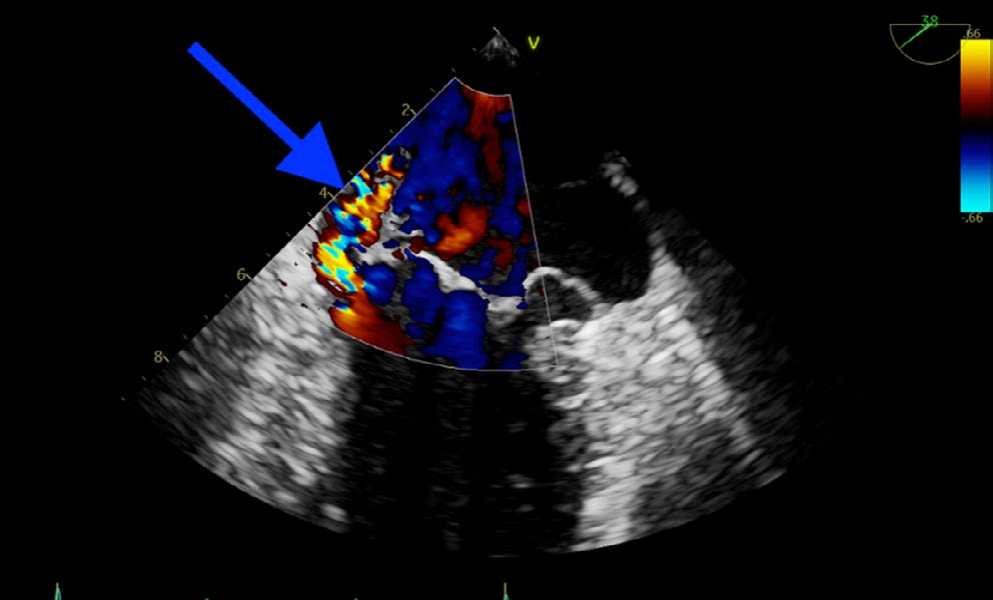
TTE with Doppler showing significant mitral regurgitation due to posterior mitral valve leaflet perforation.
